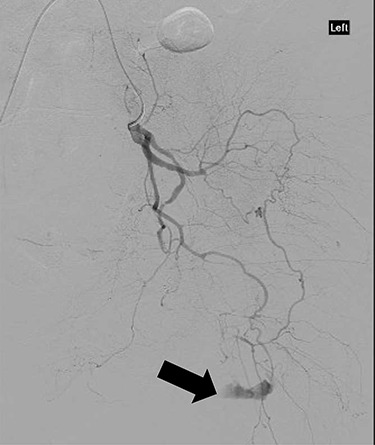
CT angiography showing extravasation of dye from muscular branch of the internal iliac artery (black arrow).
